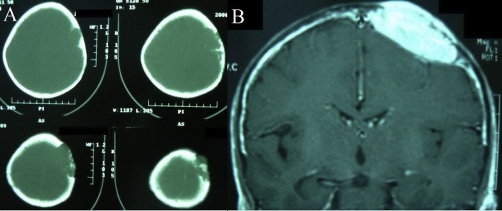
A. Axial bone window of the computed tomogram of the presented case showing a destructive osteolytic mass lesion in the left frontoparietal region. B. contrast enhanced coronal magnetic resonance imaging of the lesion, showing enhancement of the mass and no involvement of the intradural space.
